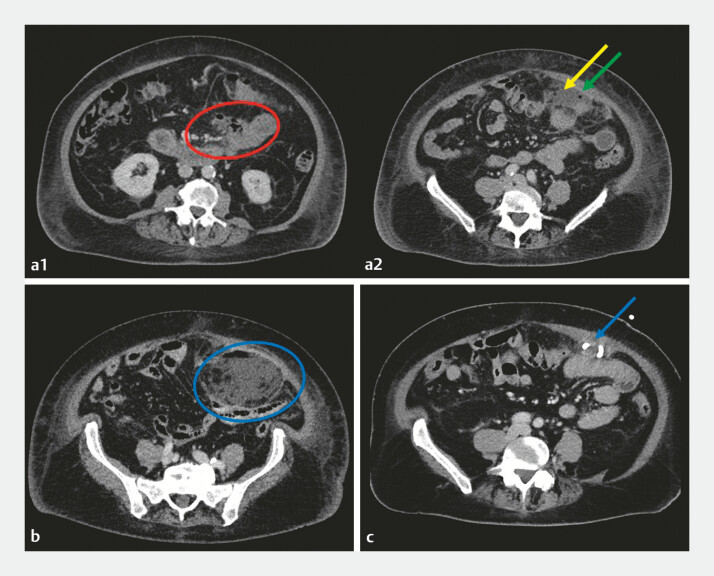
Axial CT scans of the abdomen.
